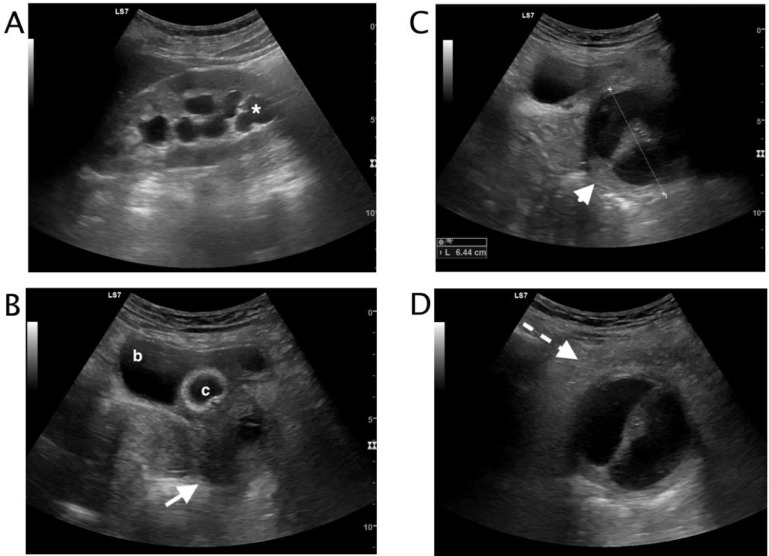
Transabdominal ultrasound (A-D) A. Mildly dilated calico-pelvic system of the left kidney (white asterix). (B) Catheterized bladder (c). Below the bladder (b) an inhomogeneous fluid filled mass was appreciable (white arrow). (C) The fluid filled mass content was slightly inhomogeneous, the mass was septate (white arrowhead) with thick wall and localized below the bladder (maximum diameter 6.44 cm). (D) Fluid perilesional suffusion and fat inhomogeneity were appreciable (dashed arrow) , a hyperechoic spot was visualized in the middle center of the septum.
